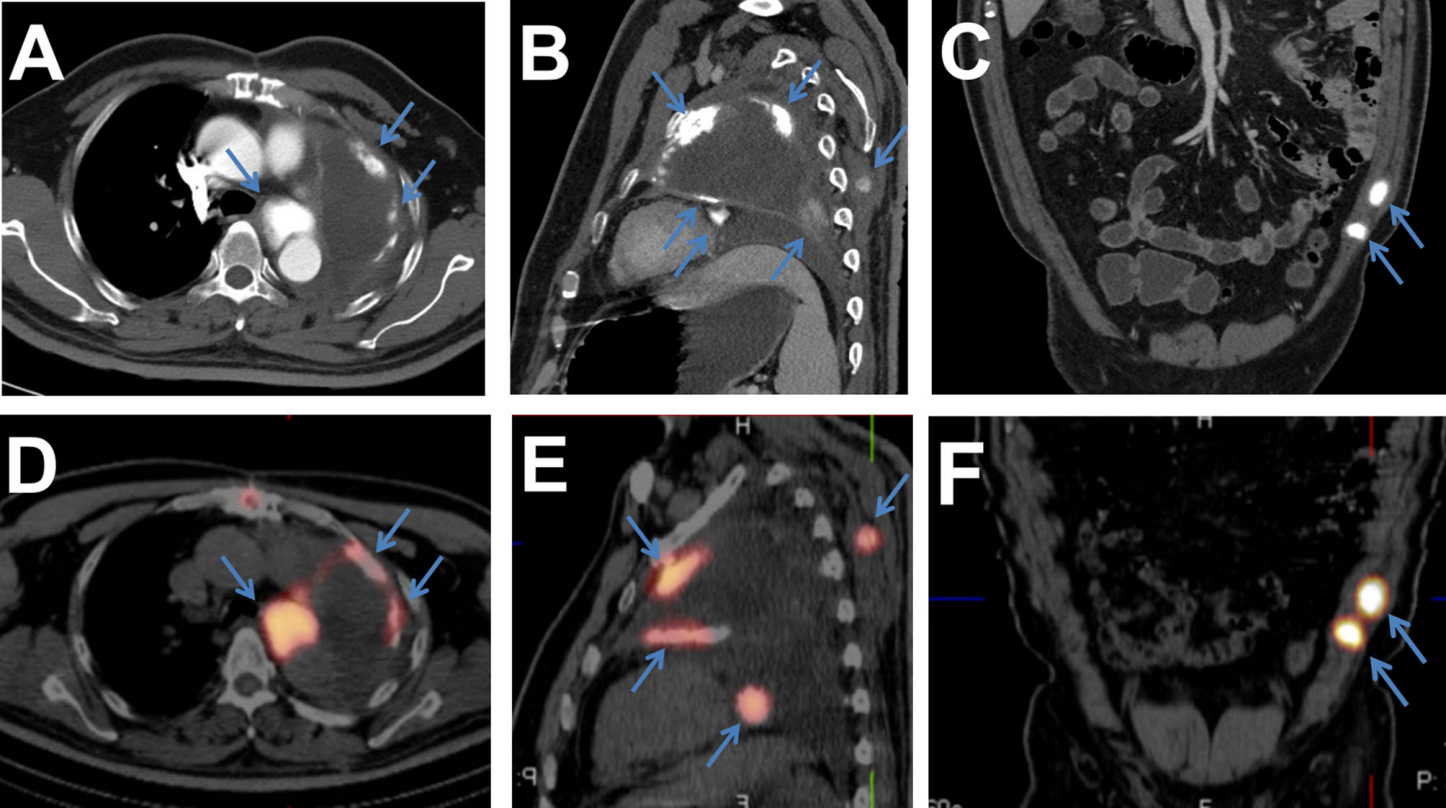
(A–C) Contrast-enhanced CT scan showing that the tumor recurred in the left lung hilum with multiple metastases in the left pleura and somatic muscles (blue arrows). (D–F) 99mTc-MDP SPECT/CT images showing the areas (blue arrows) with high concentrations of radioactivity, which were consistent with those on the CT scan.
